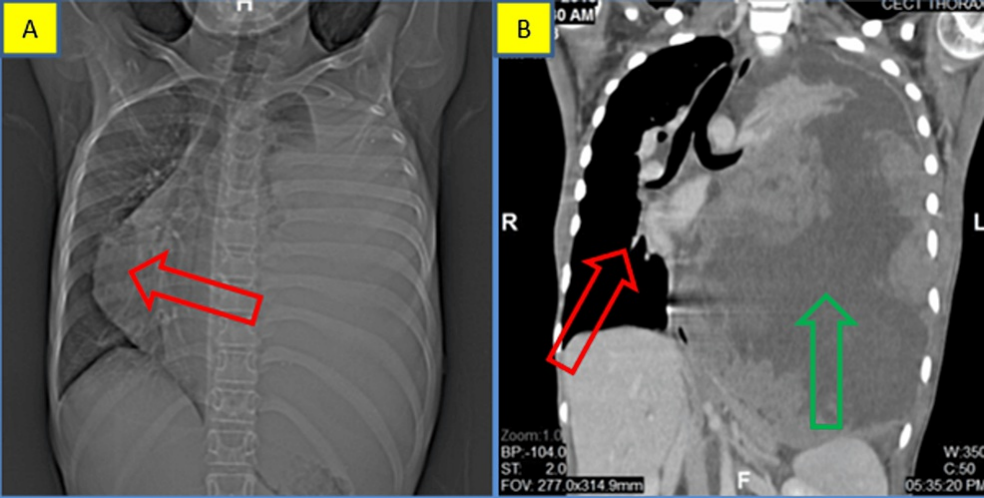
Case 1: radiological features A. Chest radiograph showing an opaque left hemithorax-sparing apex with a mediastinal shift to the right side (red arrow). B. Coronal reformation of computed tomography (CT) showing the mass filling the left lung (blue arrow) and pleural space, displacing the heart to the right side (red arrow).
